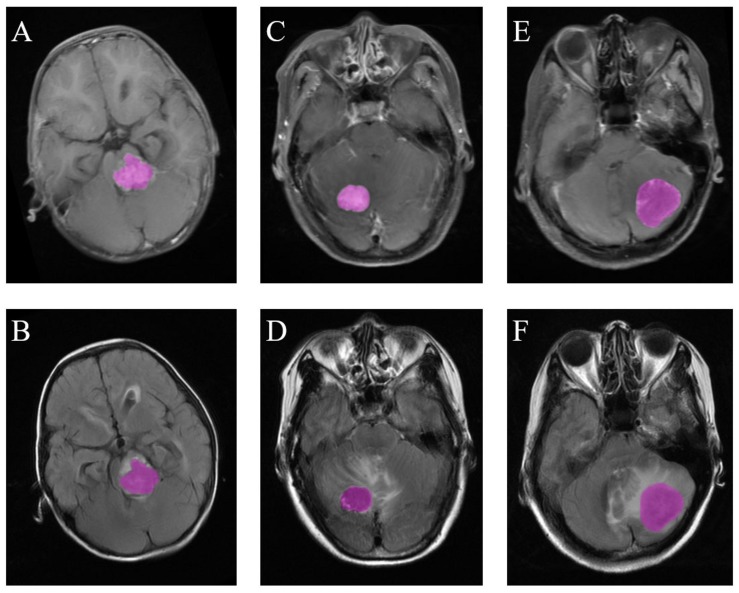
Examples of ROI delineation in the three types of tumors. (A) Medulloblastoma on T1C images. (B) Medulloblastoma on FLAIR images. (C) Hemangioblastoma on T1C images. (D) Hemangioblastoma on FLAIR images. (E) Brain metastatic tumor on T1C images. (F) Brain metastatic tumor on FLAIR images.
